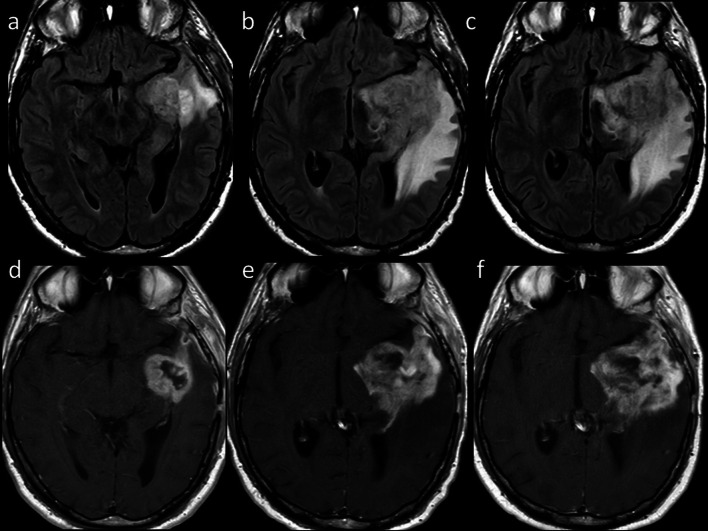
True progression. 53-year-old man with glioblastoma treated with surgery, ChT and RT. Axial FLAIR (upper row) and contrast-enhanced T1-weighted (lower row) 1 month after surgery (a, d), and 1 (b, e) and 4 (c, f) months after ChT/RT showed continuous increasing in signal changes and enhancement of the lesion
